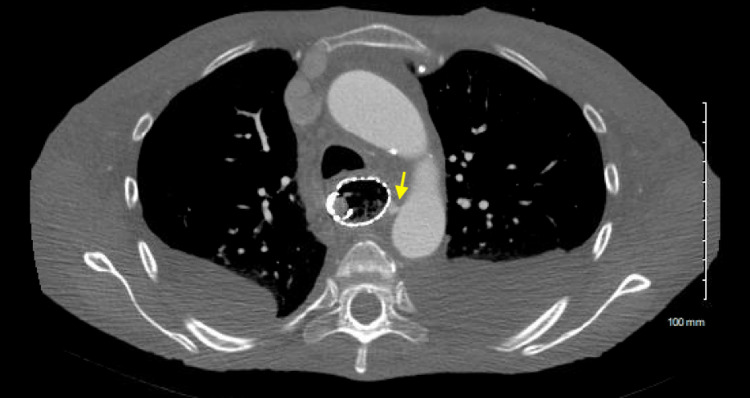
CT angiogram showing punctate aortoenteric fistula extending from the proximal descending aorta to the proximal esophagus (yellow arrow). This fistula was covered by the existing esophageal stent, without overt extravasation into the esophageal lumen
